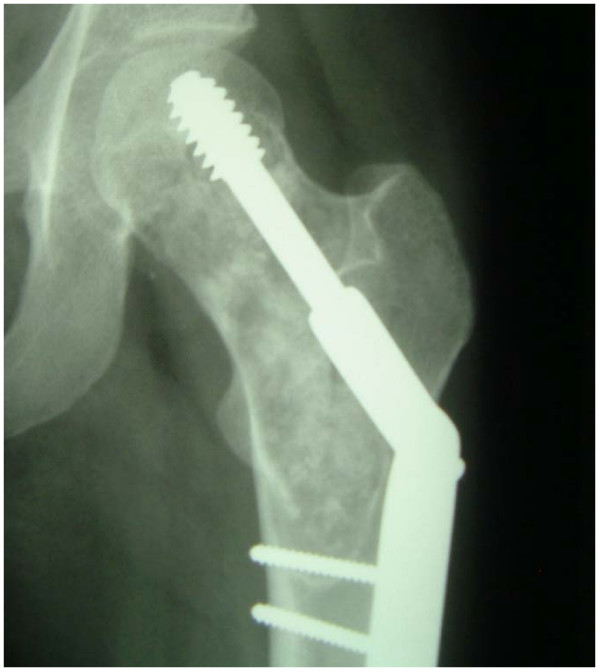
X-Ray, postoperative: spongiose plastic surgery and internal fixation (DHS).
